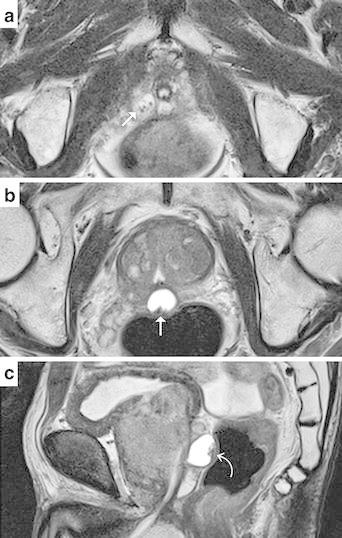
Prostate MR images. Axial T2-weighted images (a, b) showed two cystic lesions with punctate low signal intensity spot inside (→). Saggital T2-weighted images (c) demonstrated the cystic lesion at the midgland level was protruding from the prostate ()
